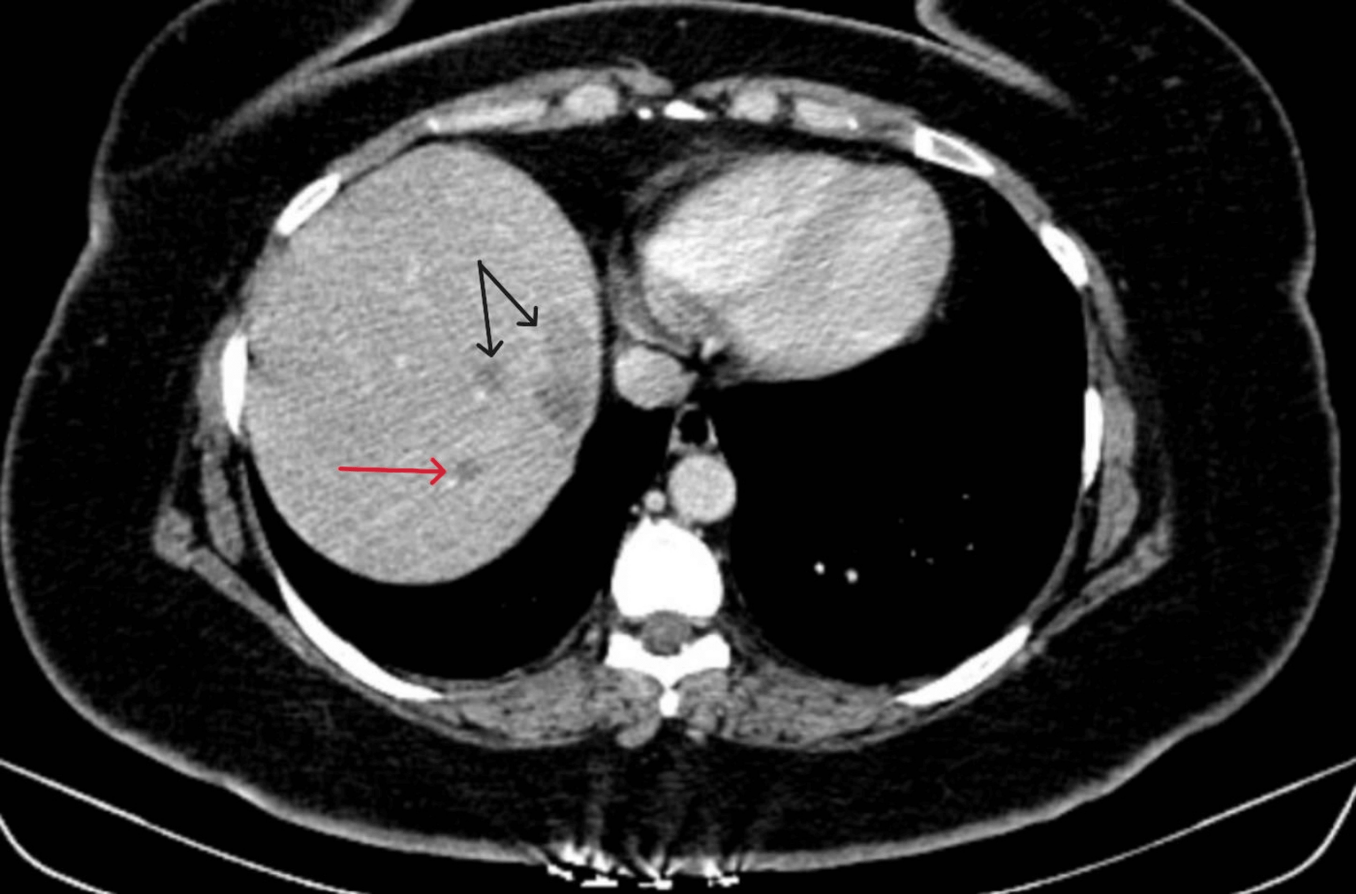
Contrast-enhanced computed tomography scan hepatic venous phase axial cut showing lesions in segments 7 (red arrow) and 8 (black arrows) of the liver.
